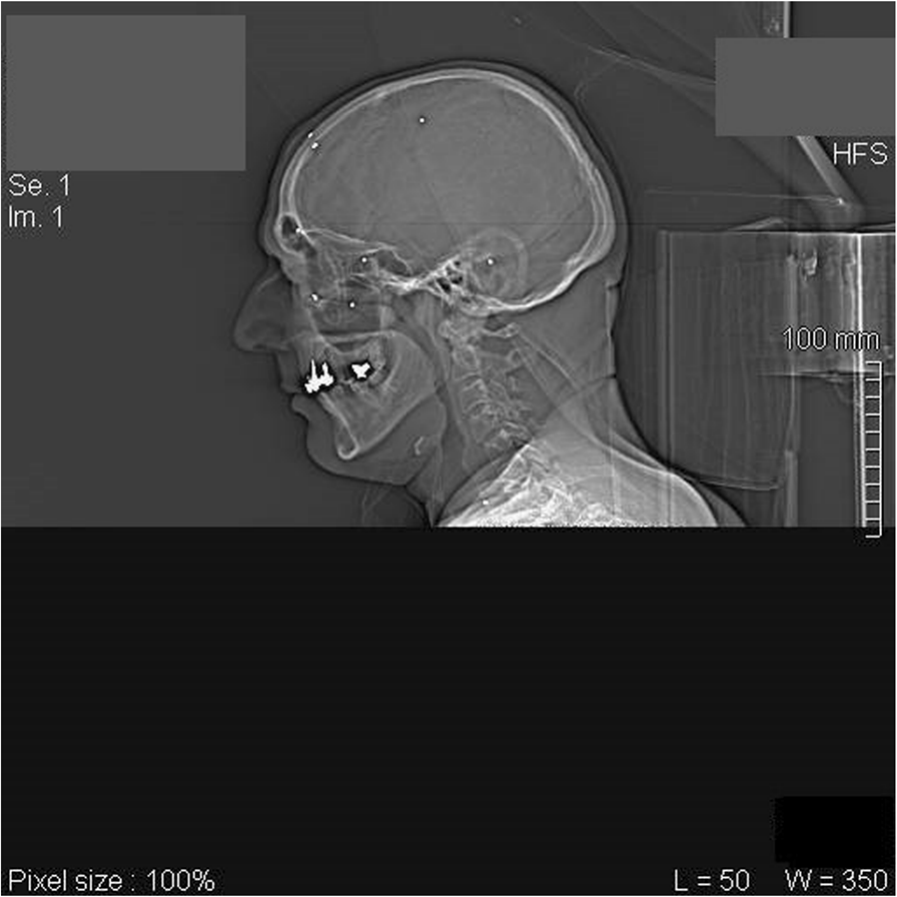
CT scan Sagittal Recontruction: head localization of numerous lead pellets
